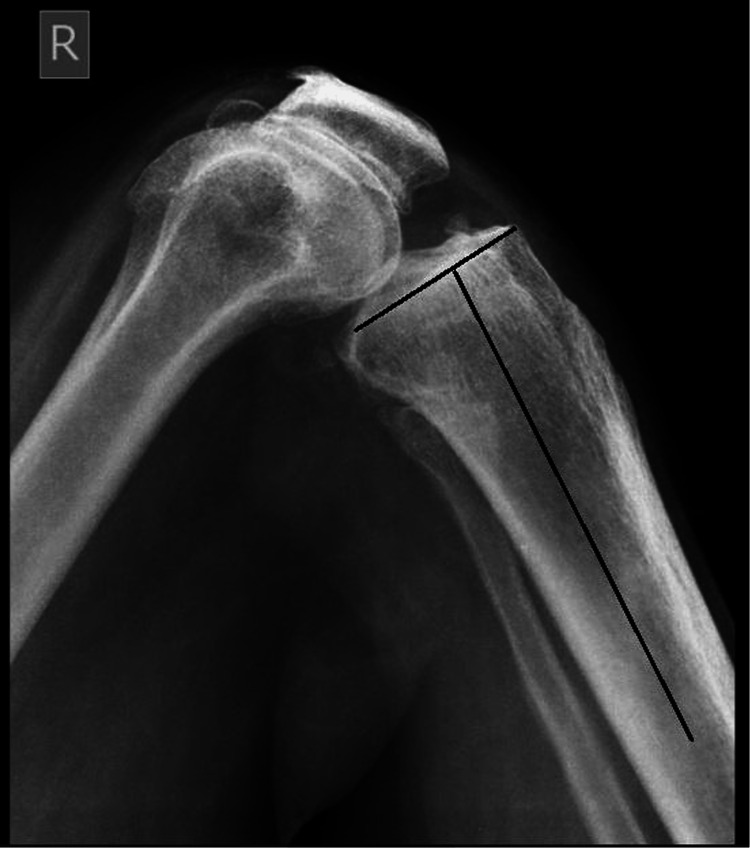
Measurement of preoperative tibial slope from the lateral view of the X-ray.
